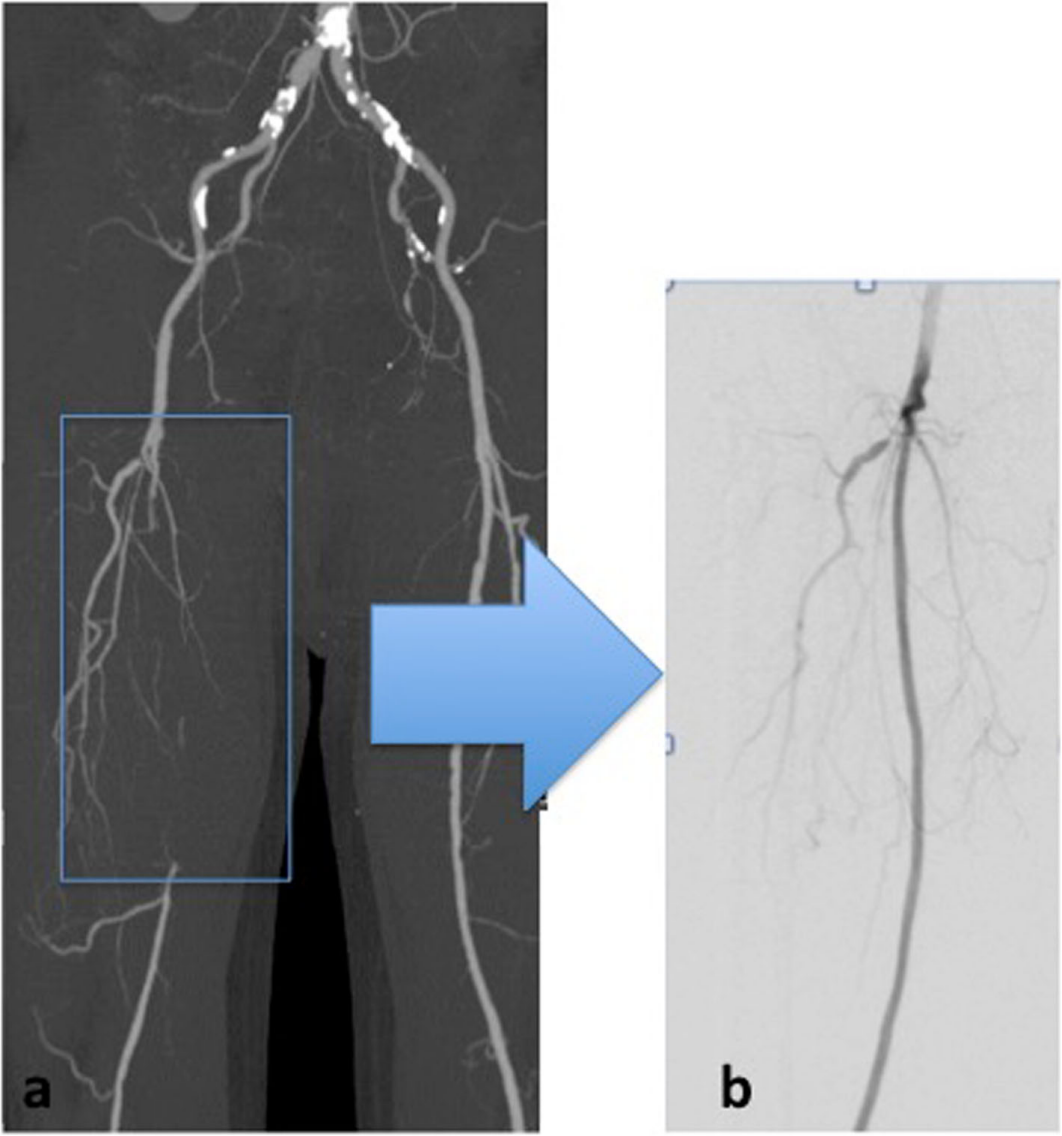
Imaging of the superficial femoral artery occlusion lesion before and after endovascular therapy. a Preoperative computed tomography angiography revealing the superficial femoral artery occlusion lesion. b Post-stenting angiography revealing no dissection
